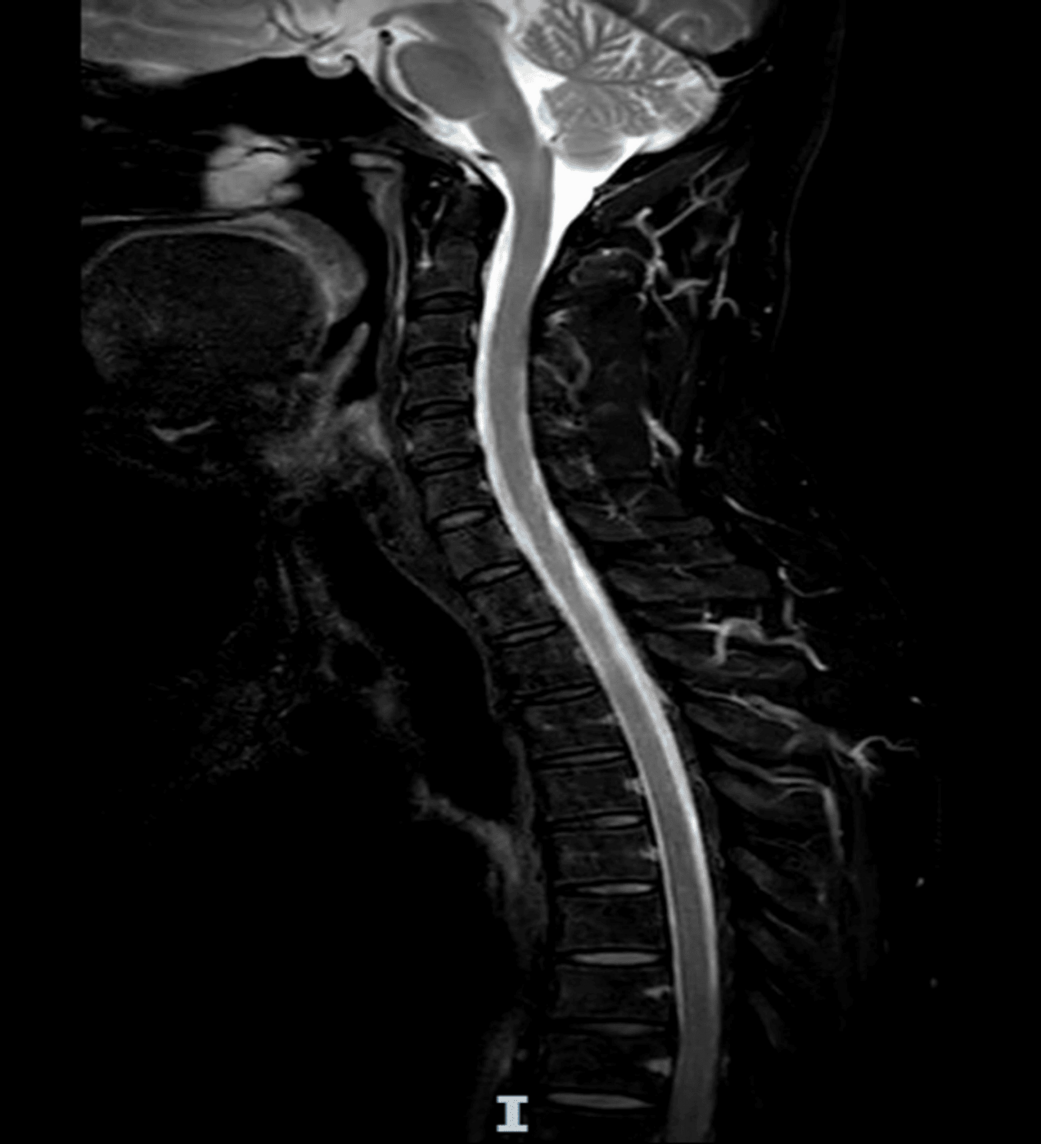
MRI of the whole spine MRI: magnetic resonance imaging
